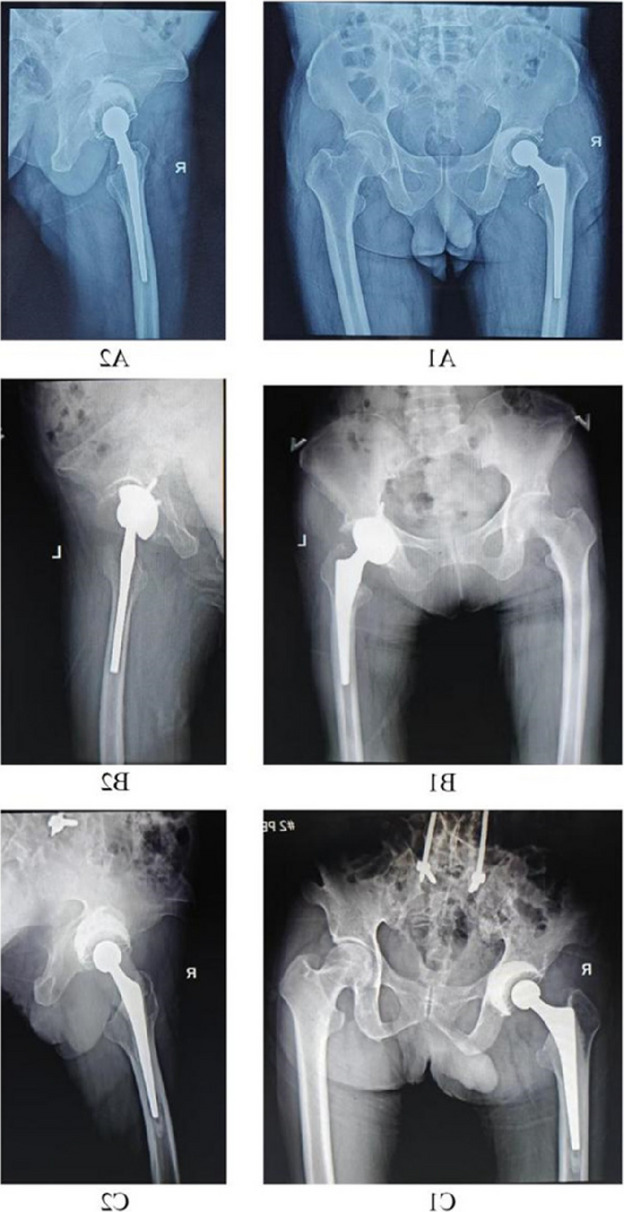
Postoperative X-rays of patients, patient 1 (A1 ~ A2), patient 2 (B1 ~ B2), and patient 3 (C1 ~ C2)
